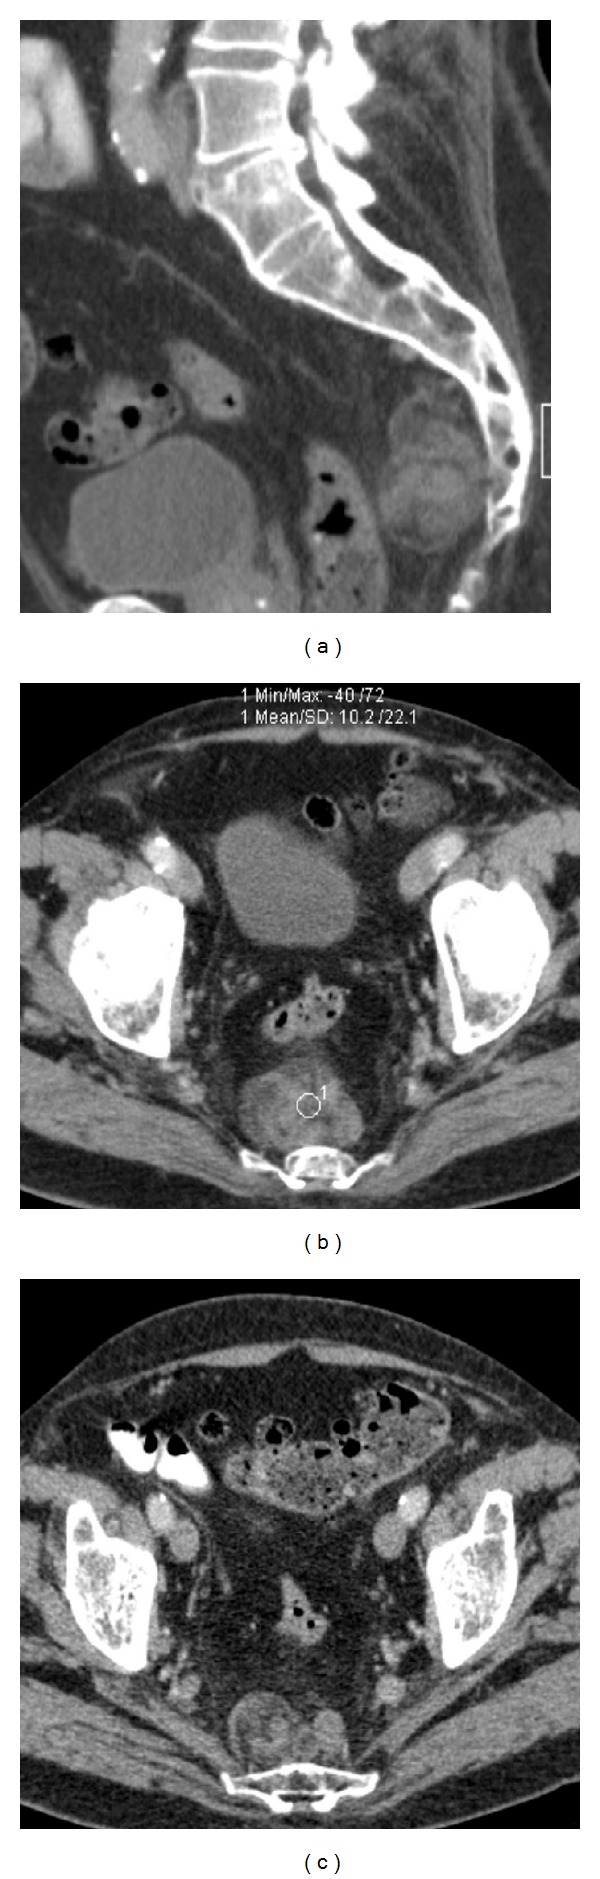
84-year-old male with presacral myelolipoma. Sagittal (a) and axial ((b), (c)) contrast-enhanced CT images show the presacral mass, spanning from S3 to S5. It presented lobulated contours and a heterogeneous appearance, due to an admixture of soft-tissue and fat elements. The latter had attenuation values of about −40 HU. The mass was located in the retrorectal space and adhered to the sacrum, posteriorly.
